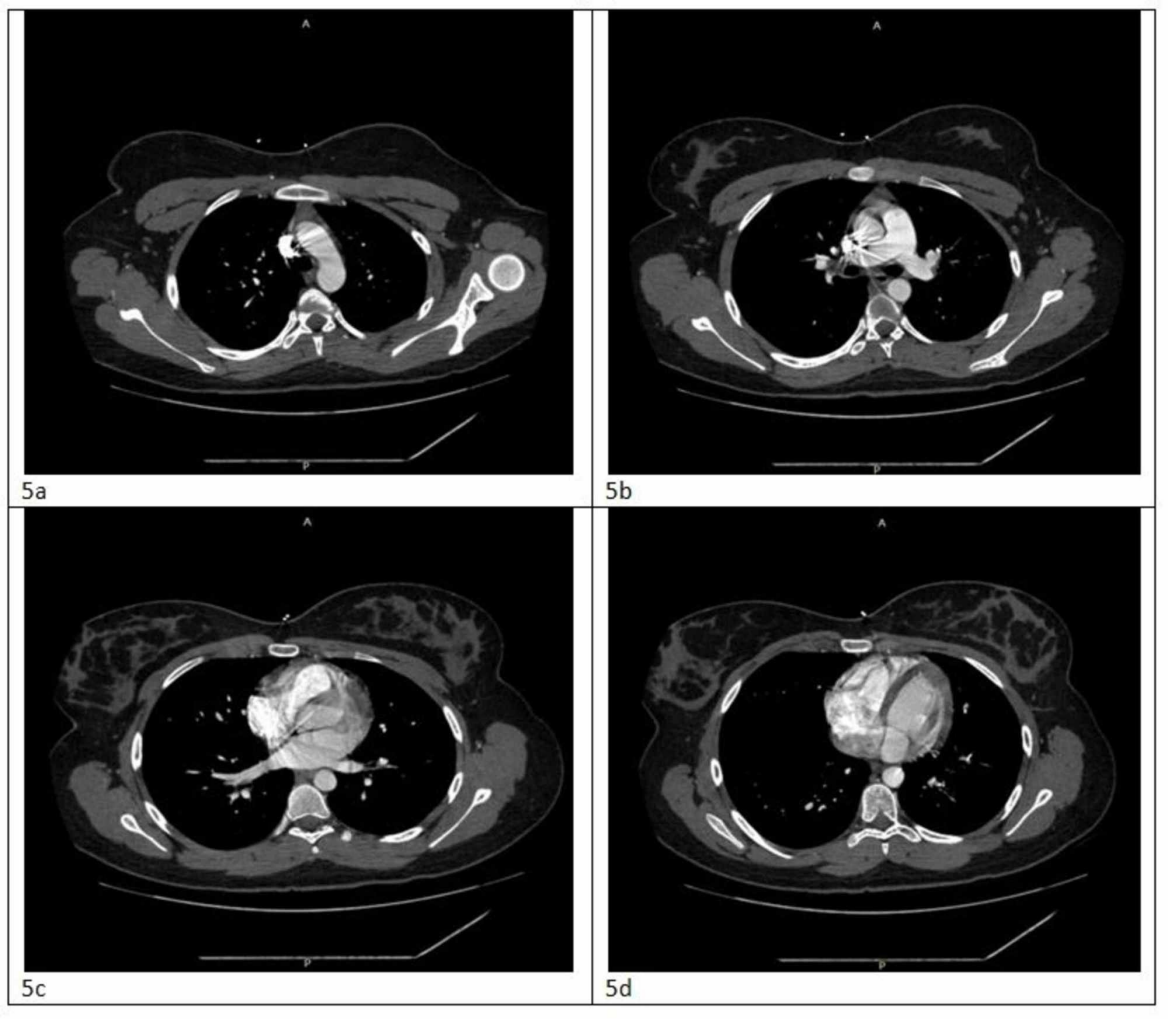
CT angiography of chest PE within normal limits (a-d) Views of the chest in different transverse planes (top to bottom) PE, pulmonary emboli
